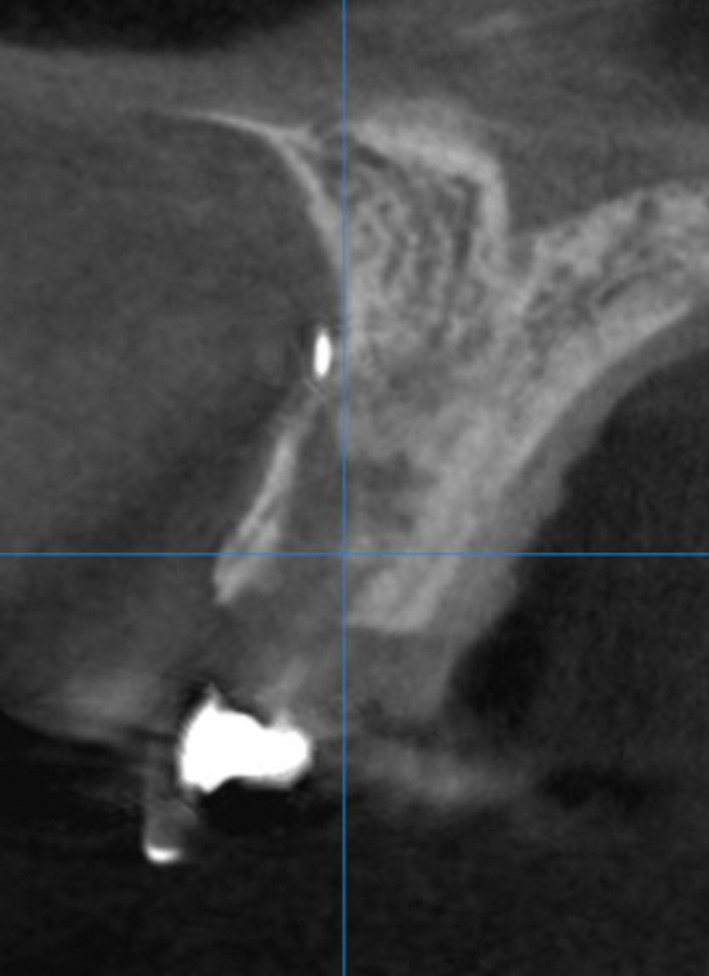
CBCT scan before implant placement into region of #11
